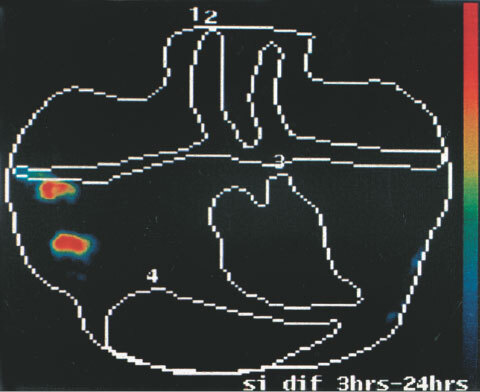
Patient BC 1, Tc-99m hHMFG-1 radioimmunoscintigraphy. Positive right axillary nodes and breast cancer.
